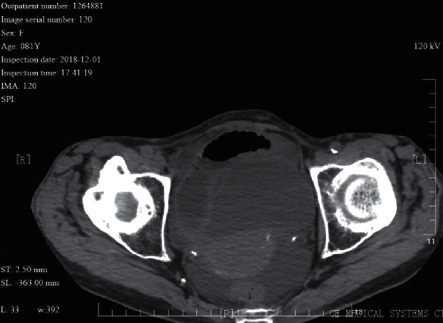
An 81 year-old female patient with internal hernia of the small intestine. The CT image shows incarcerated intestinal canal and wall pneumatosis. Ischemic necrosis of the incarcerated intestinal canal was seen during surgery.
